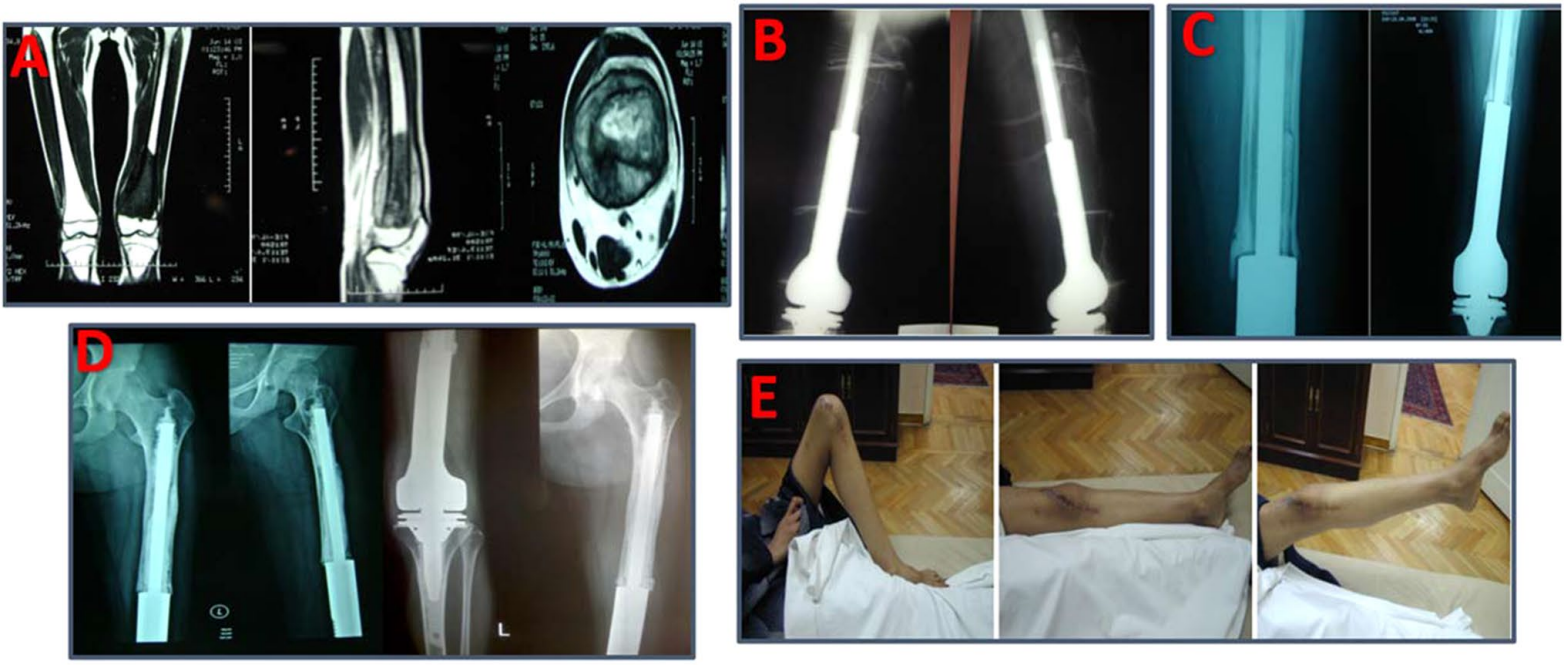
15 years old female with left distal femoral osteosarcoma and CT chest was free. A Pre-operative MRI. B Post-operative X-ray. C 6 years follow-up showing loosening of the femoral stem. D Post-revision X-rays. E Final range of motion with MSTS score of 24 (s years after the revision)
